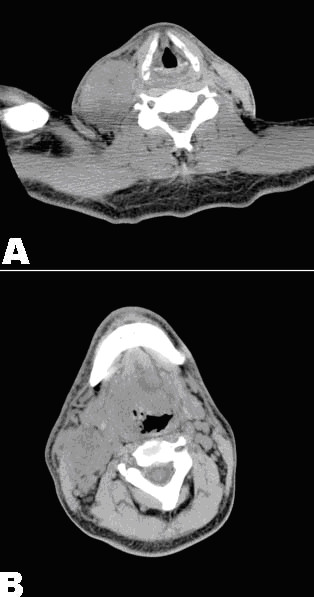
Images of computed tomography (CT) without contrast. A. Lesion at the base of tongue (indicated by an arrow); B. Conglomerate mass invading the right sternocleidomastoid muscle (indicated by an arrow).
